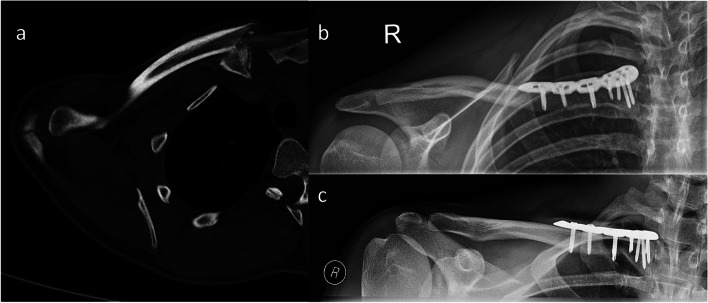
a Axial Computed tomography (CT) image of a medial end clavicle fracture preoperatively. b and c present x-rays in two planes after surgical fixation of the fracture using an anatomically preformed locking compression plate (LCP, Synthes, Umkirch, Germany)
